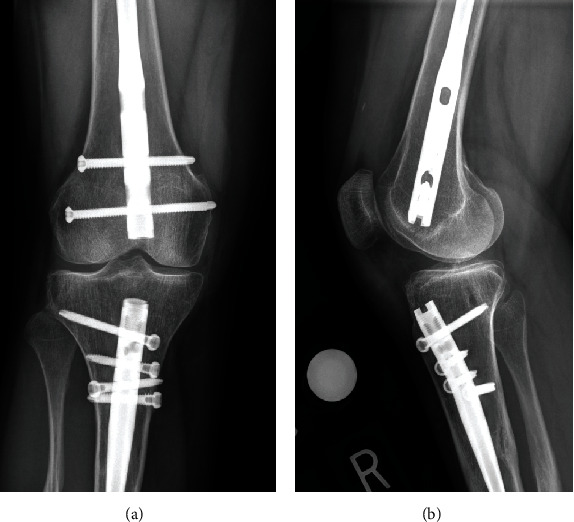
Three-month postoperative radiographs confirming complete resection of the anterior intra-articular heterotopic ossification: (a) anterior-posterior image; (b) lateral image.
